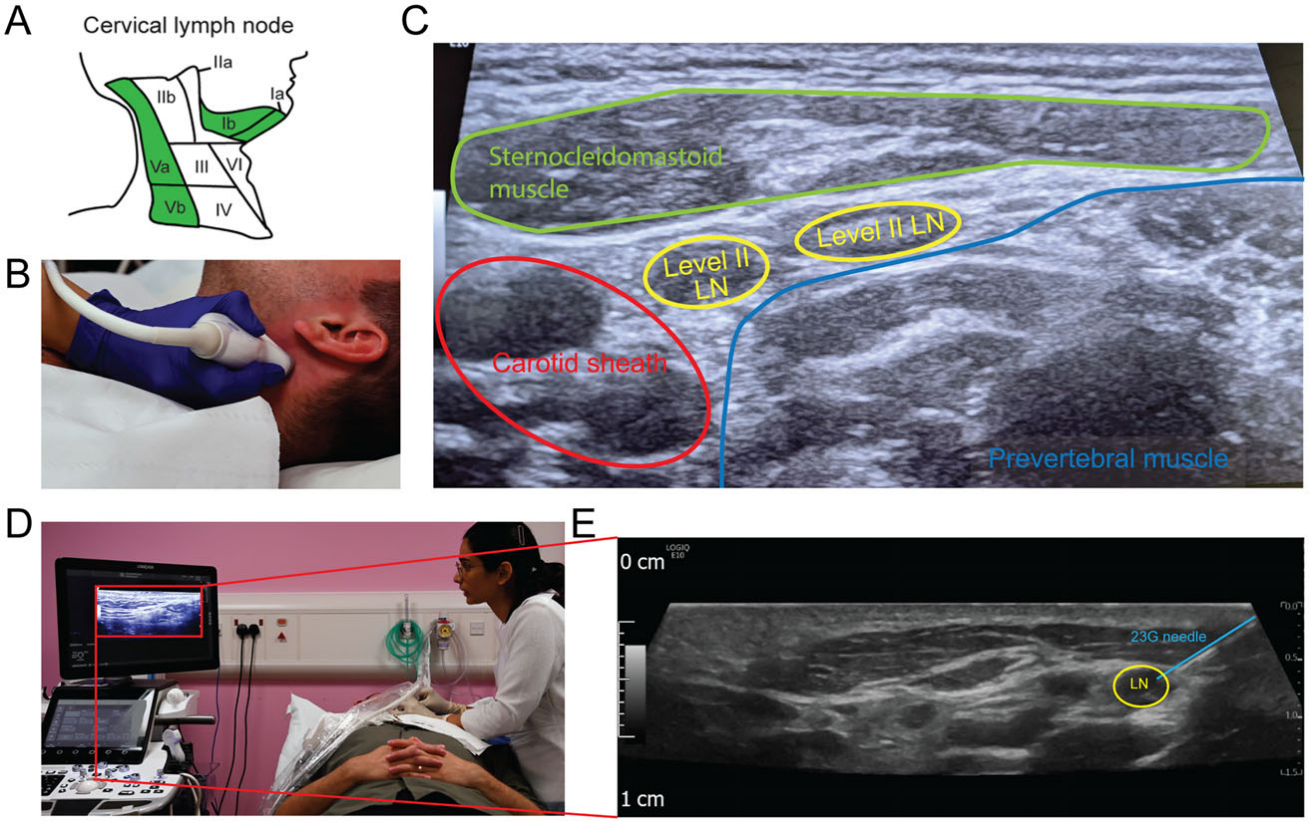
Cervical LN sampling. (A) Anatomic localization of the cervical LNs within the neck. Our prior sampling has been primarily from level 1 and 5 LNs, highlighted in green. Reproduced from Al‐Diwani et al. (2022). (B) Locating an accessible cervical LN. (C) Ultrasound image of the neck with detailed anatomic annotation of key major structures. (D) Sampling of the cervical LN under ultrasound guidance. (E) Ultrasound image of the neck with the LN and the FNA needle highlighted.
